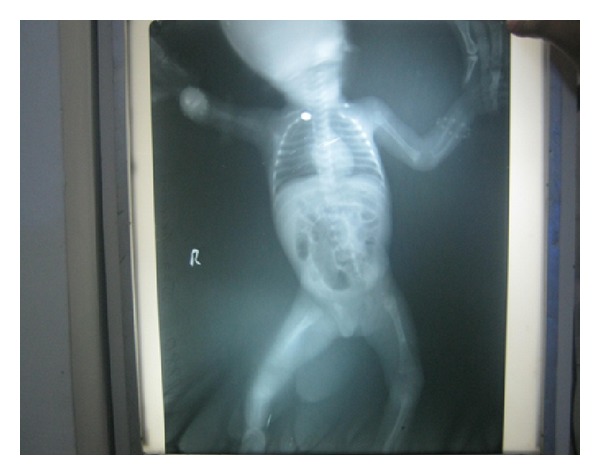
Radiograph showing lower end of humerus (periostitis) and proximal ends of radius and ulna (metaphysitis).
